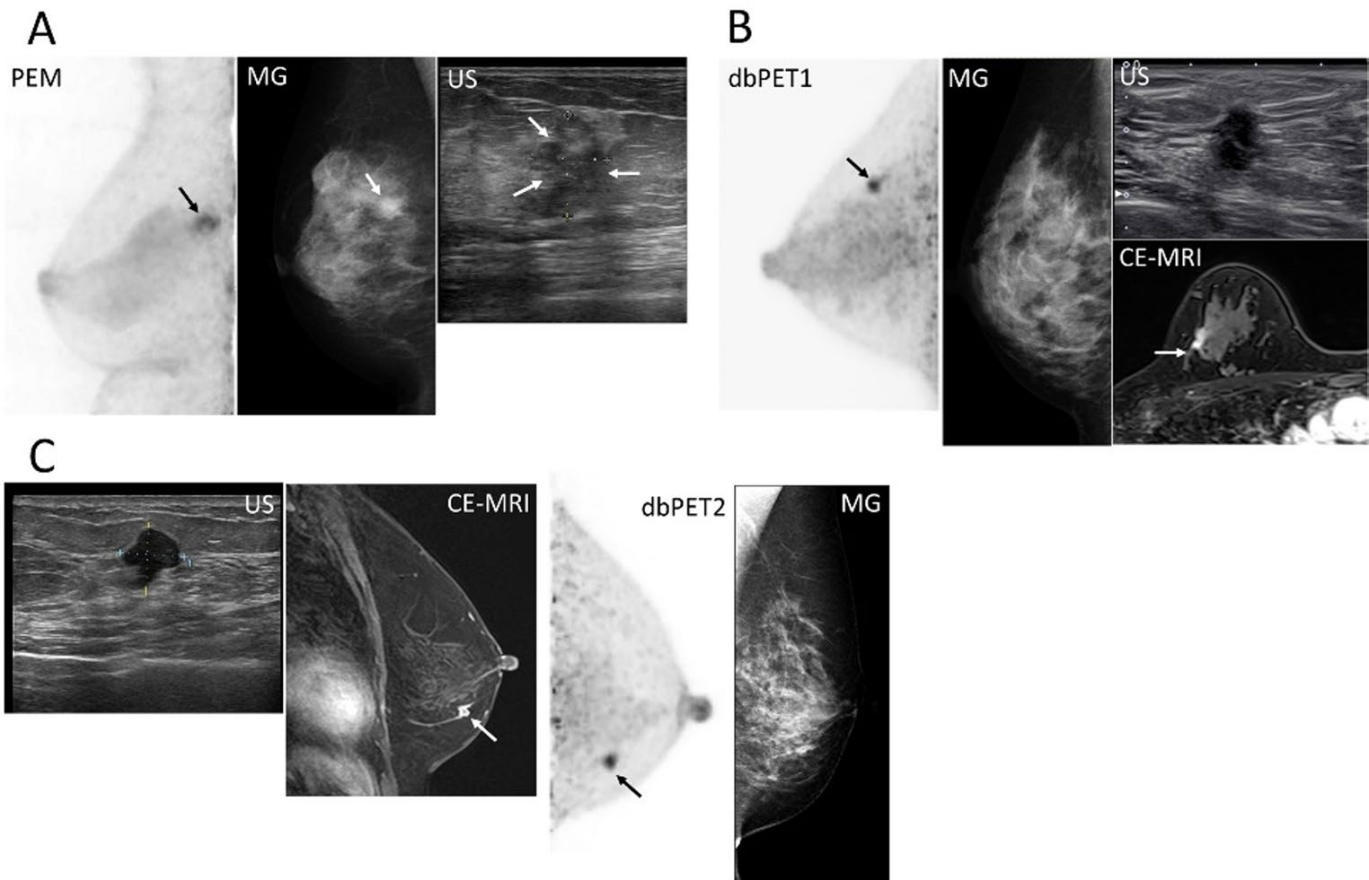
Representative breast-specific PET images for a mass uptake slightly closer to the chest wall and inter-modality differences. The primary breast cancer lesions are indicated by arrows. All PET images are displayed using an SUV scale ranging from 0 to 5. (a) A 44-year-old woman with invasive ductal carcinoma (IDC, Luminal A). (b) A 45-year-old woman with IDC (Luminal B). dbPET1, mammography (MLO), ultrasonography, and contrast-enhanced MRI. The arrow marks the primary lesion; mild peripheral noise is visible near the chest-wall side of the FOV. (c) A 56-year-old woman with IDC (Luminal B). dbPET2, mammography (MLO), ultrasonography, and contrast-enhanced MRI. The primary lesion is indicated by an arrow; a slight peripheral noise increase is observed. Abbreviations: PET, positron emission tomography; PEM, positron emission mammography; MG, mammography; MLO, mediolateral oblique; US, ultrasonography; IDC, invasive ductal carcinoma; FOV, field of view; dbPET, dedicated breast PET; CE, contrast-enhanced
